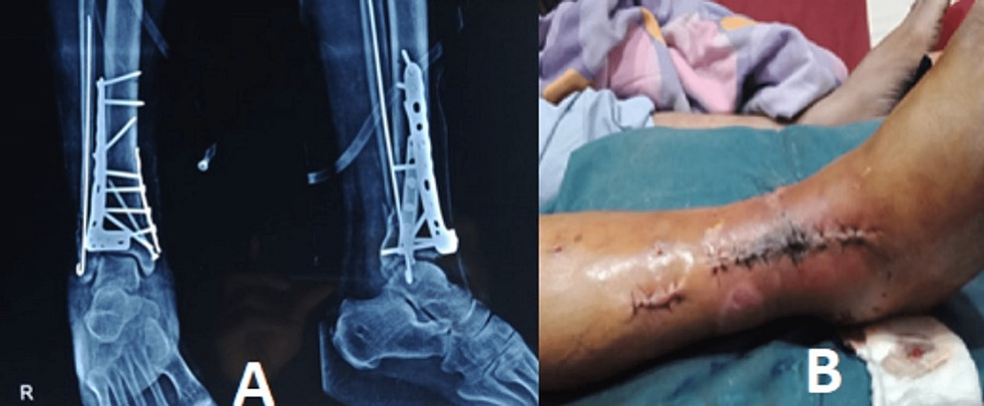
Open reduction and internal fixation X-rays and clinical image of the wound (Step 2) A: Post-operative X-rays in anteroposterior and lateral views B: Clinical image of post-operative day two surgical site
